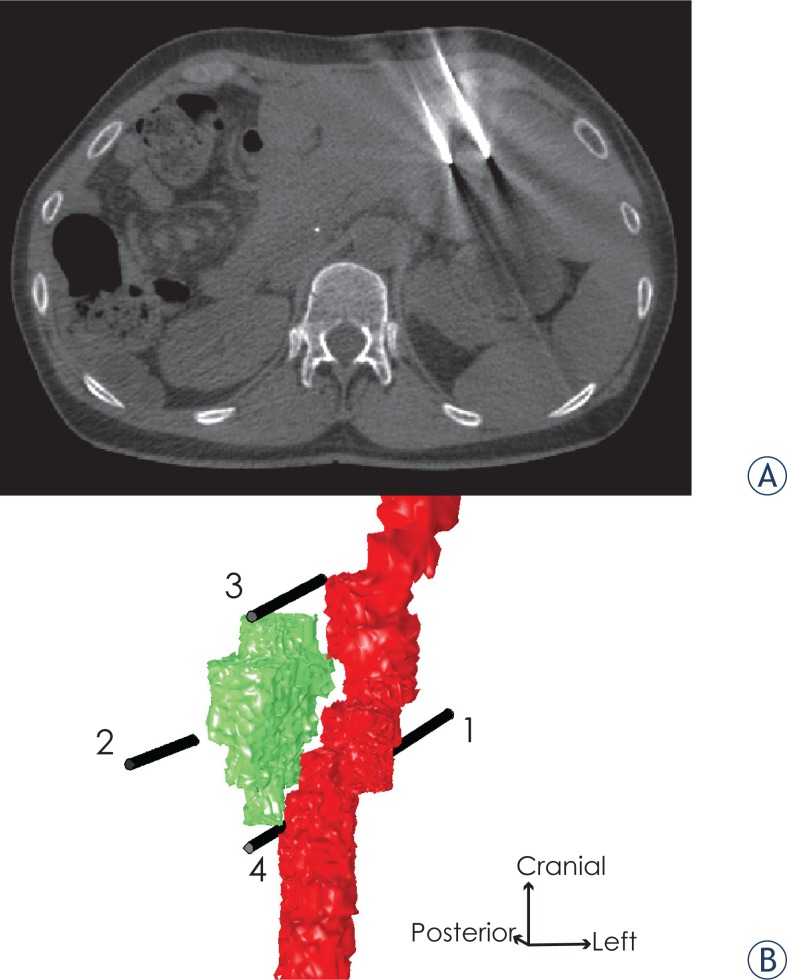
(A) CT image showing position of two of the electrodes (electrodes 1 and 2) inside the liver. (B) 3-D model showing the positioning of the electrodes in the model relative to the tumor (green) and the left liver vein (red). The liver, which was also included in the computational model is removed from the image for clarity, since it is completely encompassing the region of interest. The numbers identify each electrode.
